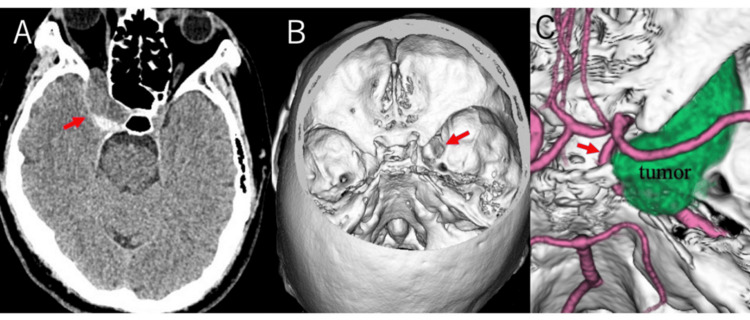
Preoperative images. A: Head computed tomography (CT) showing a cystic lesion within the right cavernous sinus (red arrow). B: The tumor enlarged the superior orbital fissure (red arrow). C: The tumor was adherent to the lateral wall of the cavernous segment of the internal carotid artery (red arrow).
